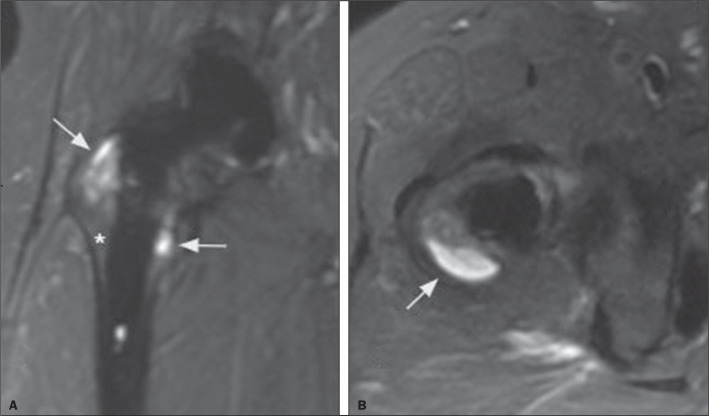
Osteolytic and osseointegrated zones in THA on MRI (MAVRIC-SL). A:
